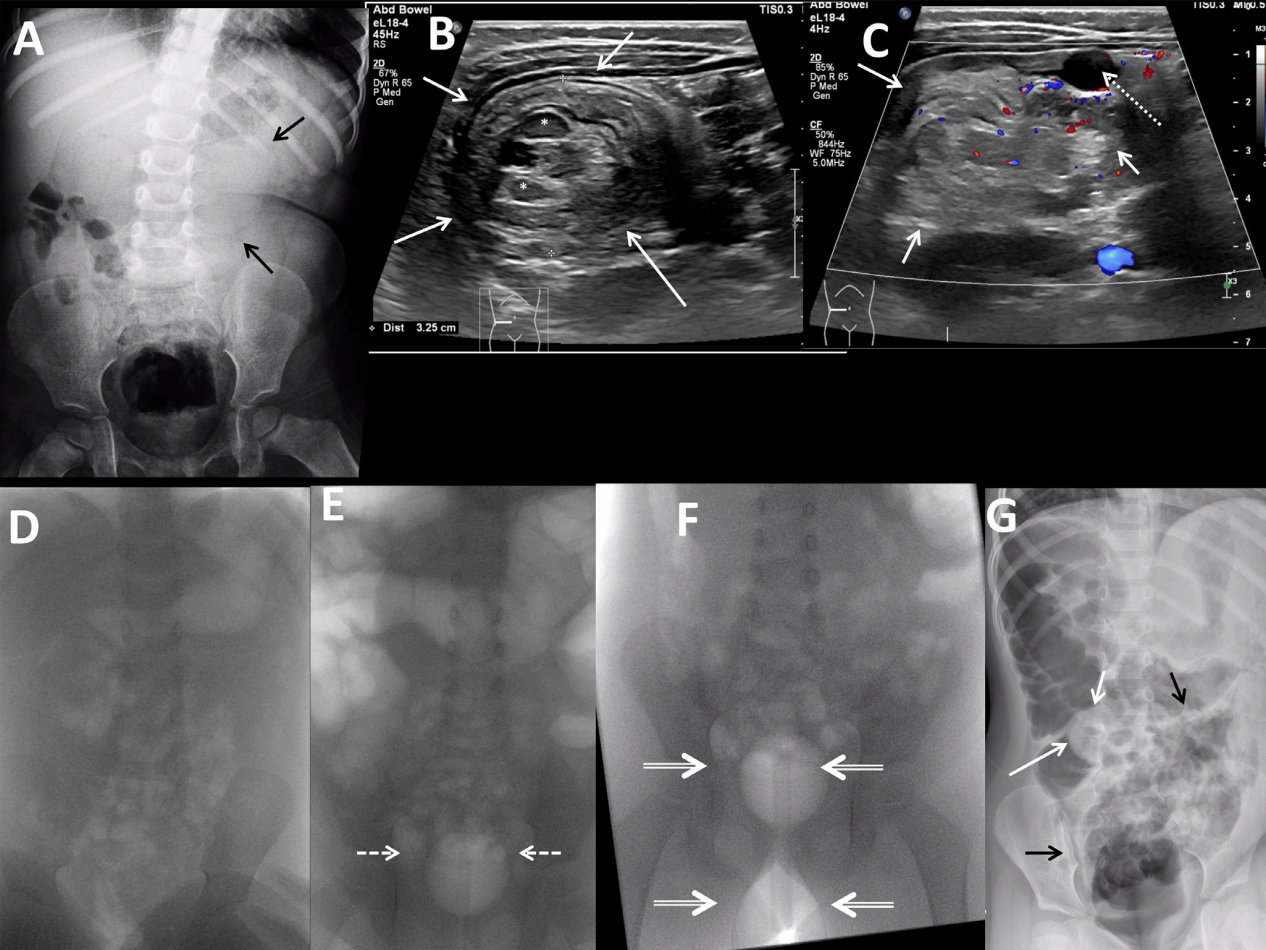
Two-year-old female with ileocolic intussusception and unsuccessful reduction. (A) Frontal supine radiograph of the abdomen shows a suggestion of a tubular soft tissue mass in the midabomen (black arrows). (B, C) Linear transducer grayscale transverse image of the right midabomen shows a 3.2 cm diameter round mass (white arrows) containing lymph nodes (white asterisks), echogenic fat, bowel, and alternating concentric layers of echogenic tissue and internal color Doppler signal. Gallbladder noted (dotted white arrow). (D) Frontal supine fluoroscopic image of the abdomen prior to air insufflation shows minimal bowel gas. (E) Frontal supine fluoroscopic image of the abdomen with 30 mL air in 20 Fr Foley catheter balloon (white dashed arrows). All intrarectal air is displaced by the balloon. (F) Frontal prone fluoroscopic image of the abdomen with 50 mL air in internal balloon, 70 mL air in external balloon (white double arrows). All intrarectal air is displaced by the balloon. (G) Left side down lateral decubitus view after attempted intussusception reduction shows rounded filling defect at the ileocecal valve (white arrows), increased air in the cecum and central abdominal bowel loops (black arrows), and no pneumoperitoneum.
